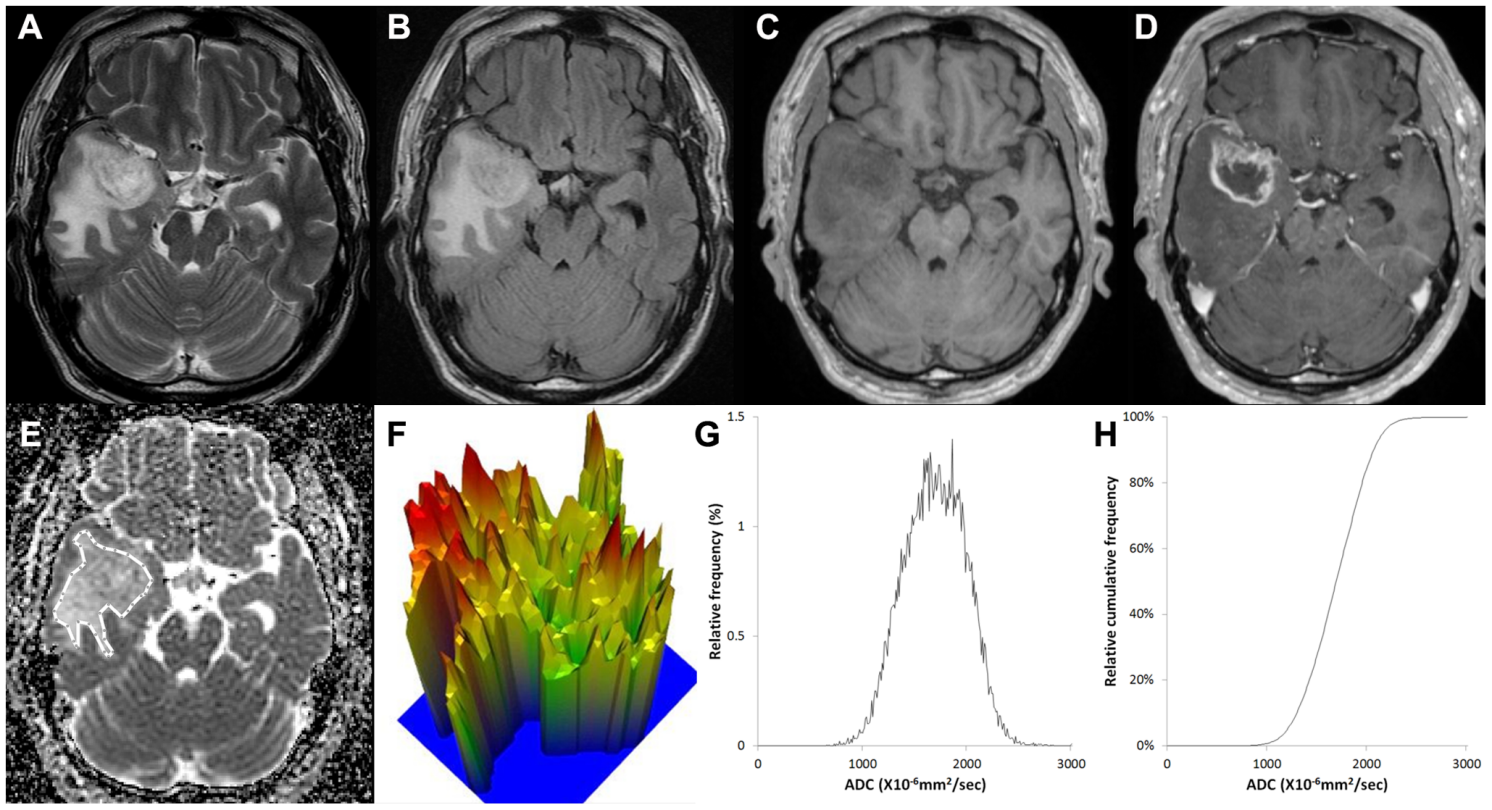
Images of a 62-year-old male with a grade IV glioblastoma. (A) T2-weighted image, (B) T2 FLAIR image, (C) T1-weighted image, (D) contrast-enhanced T1-weighted image, (E) ADC map with ROI placement, with the corresponding (E) 3-D height map of the ADC signal intensity, (G) histogram of ADC and (F) cumulative ADC histogram. The entropy value of ADC was 7.05.
